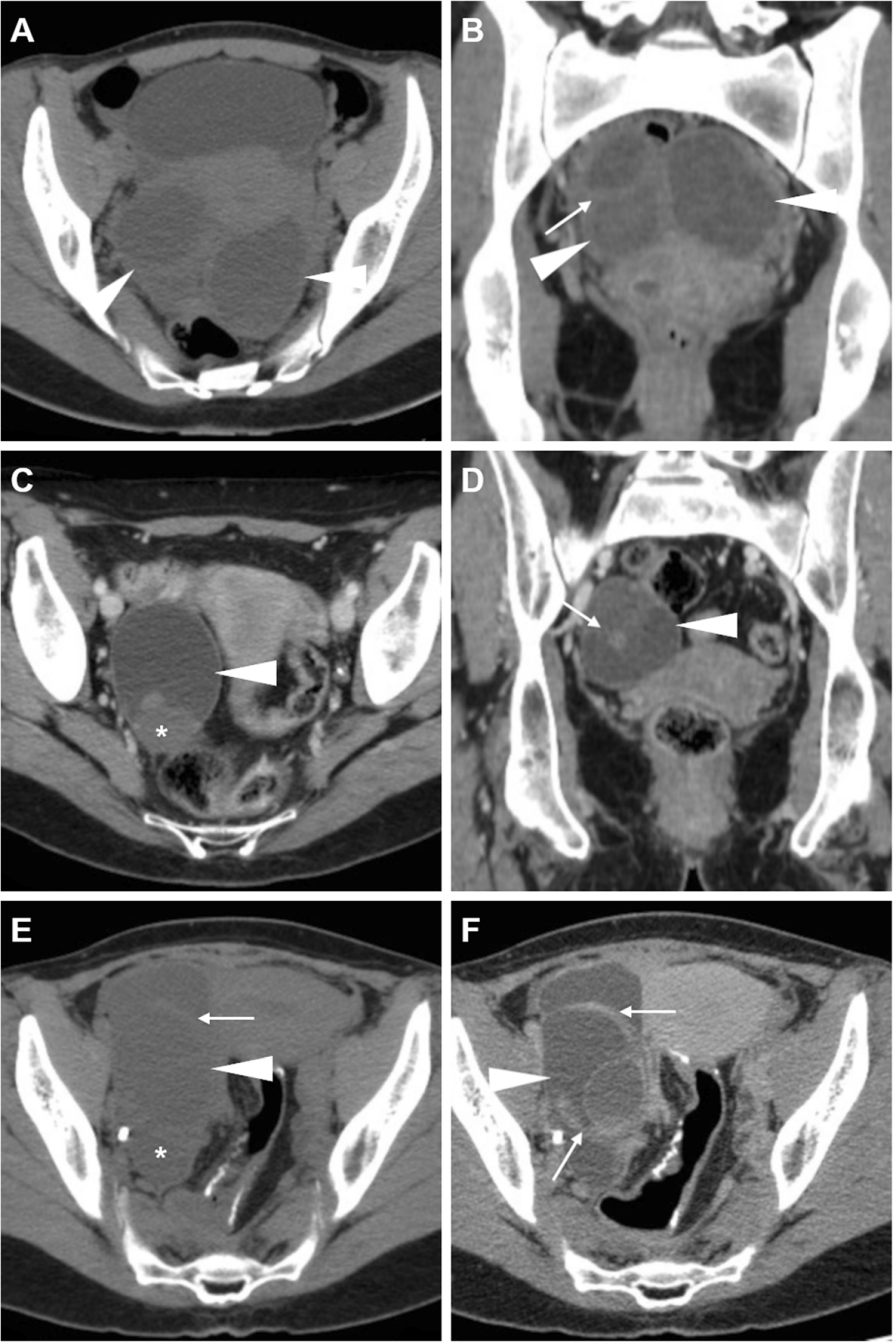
Key CT differential diagnoses of haemorrhagic ovarian cysts. a, b Unenhanced (a) and postcontrast (b) CT images of bilateral endometriomas (arrowheads) occupying the rectouterine pouch in a ‘kissing ovaries’ configuration. Note the mildly inhomogeneous attenuation and septation (thin arrow in b). c, d Ovarian carcinoma in a premenopausal woman with predominantly cystic pattern, septations (thin arrow in d) and a solid, enhancing eccentric mural vegetation (asterisk in c). e, f Unenhanced (e) and post-contrast (f) images of a postoperative collection (complex serocele, arrowheads) involving the adnexal region, showing septations (thin arrows). Note the right ureteral stent and suture at the sigmoid colon after multiple surgeries for Crohn’s disease
